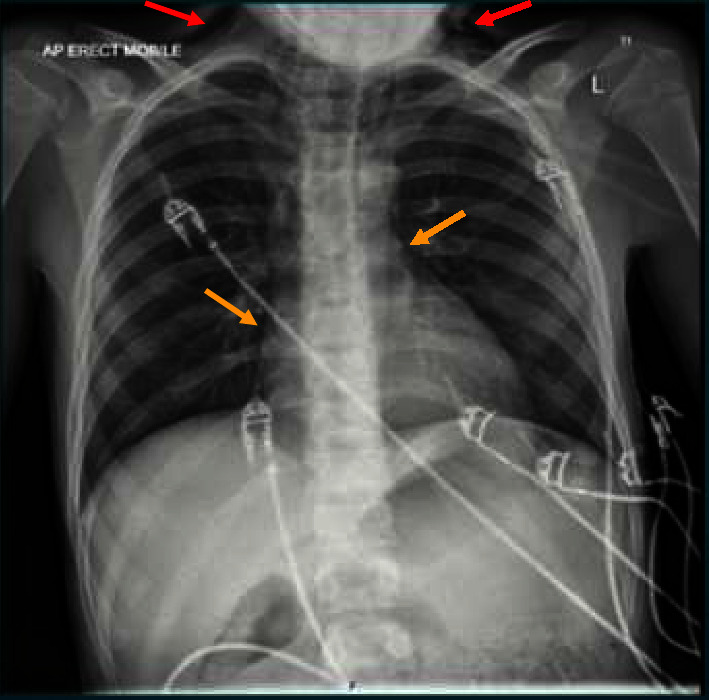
Chest X-ray AP view; red arrows point to subcutaneous emphysema in the neck and yellow arrows point to pneumomediastinum.
